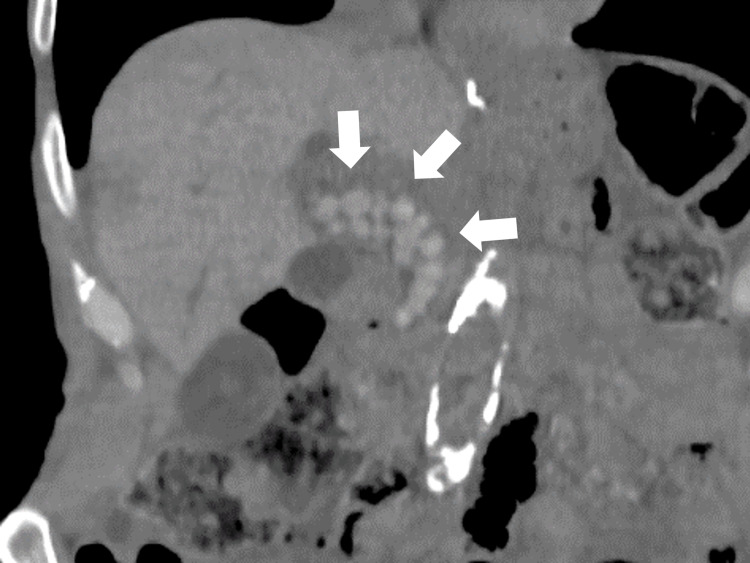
Abdominal computed tomography showing multiple common bile duct stones (white arrows)
